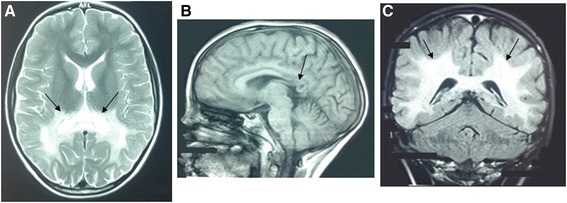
Brain MRI of the patient with high signal intensity of the white matter in axial T2, the arrows show high signal in the corpus callosum (a), low signal intensity of the white matter in sagittal T1 (b), low signal in the splenium of corpus callosum (arrow B) and diffuse high signal intensity of the white matter in coronal Flair (arrows c)
